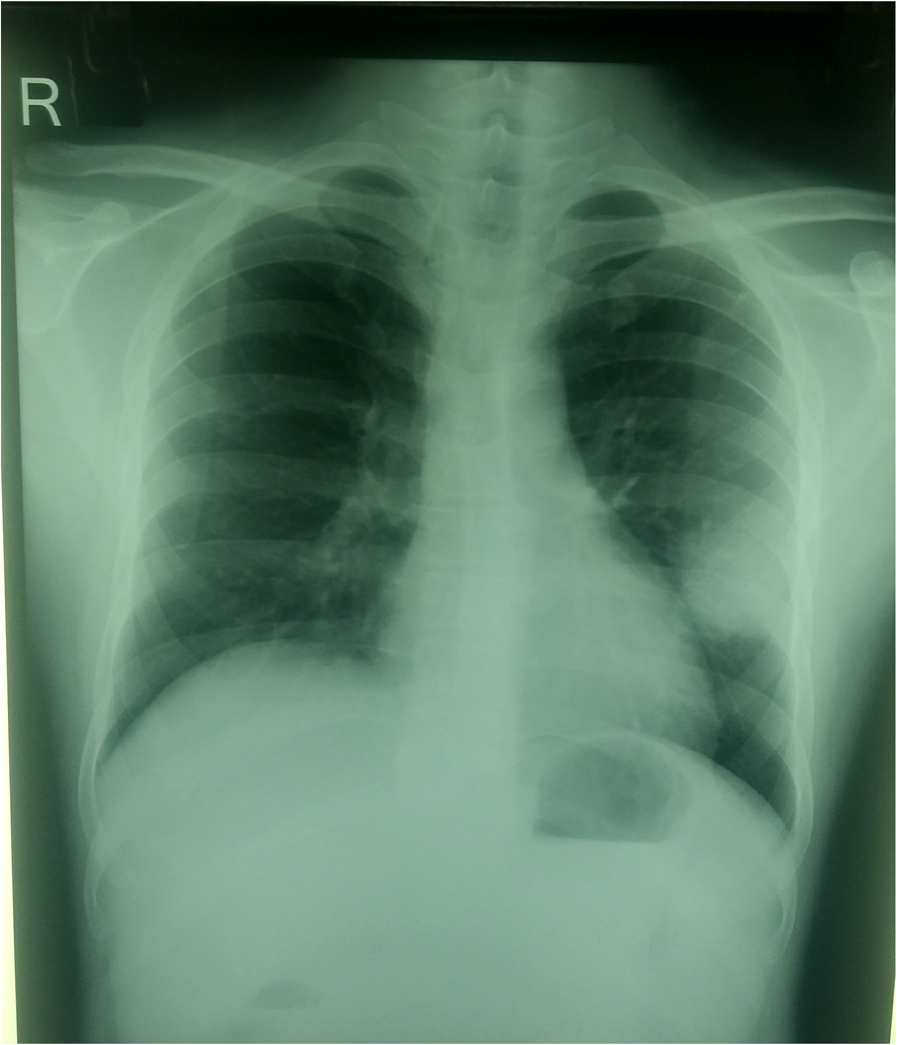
(Case I) Chest X-ray showing cavitary lesion in left lower lobe
